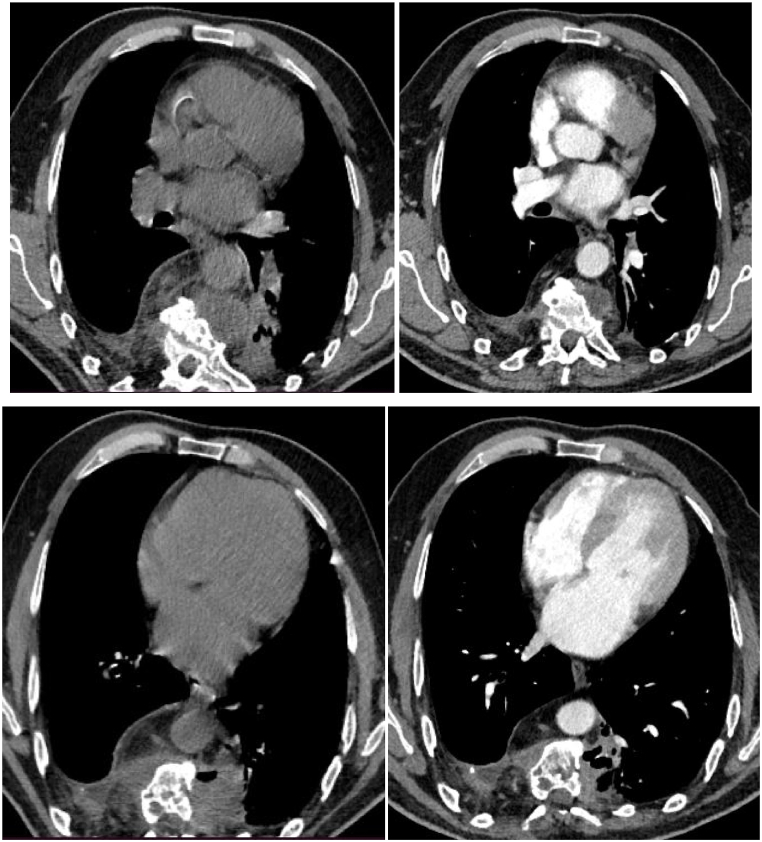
CT scan cross section at T8 vertebra and T9 vertebra (2020 on the left and 2022 on the right).
